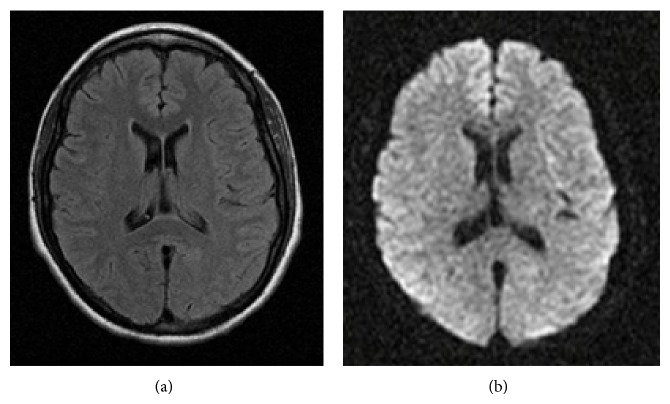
(a) MRI on FLAIR sequence showing mild diffuse subcortical edema. (b) MRI in diffusion sequence, without signal restriction.
